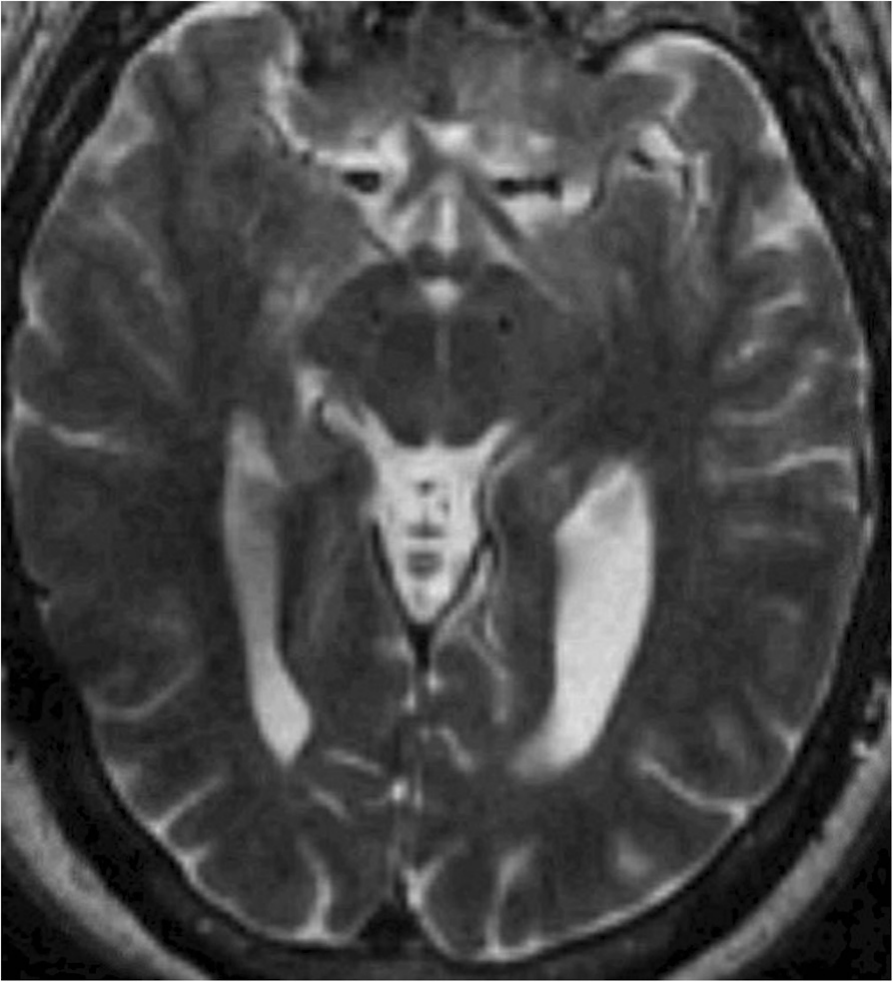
Axial T2 magnetic resonance image showing DBS lead tips in the subthalamus region, approximately 9 mm from the midline on both sides
